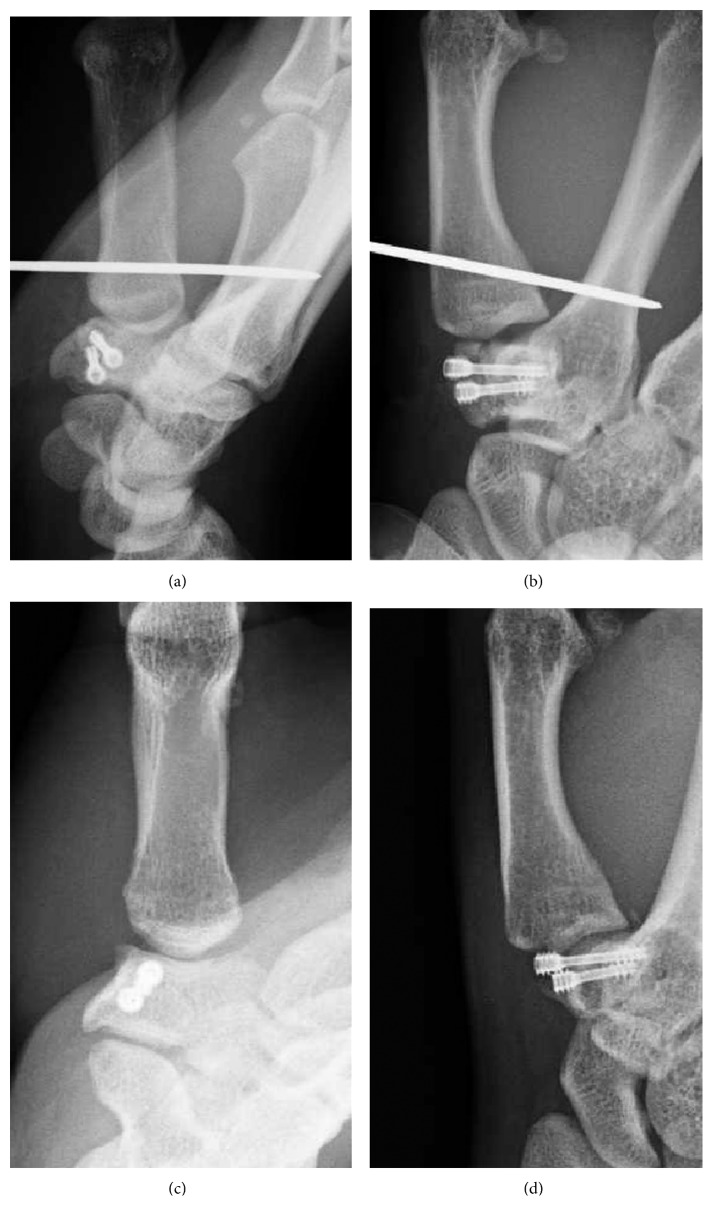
(a, b) Radiographs taken directly after surgery. Good reduction and fixation were obtained using 2 double-thread headless screws. (c, d) Radiographs taken at final follow-up, a year after surgery. A congruent CMC joint and trapezium fracture union is shown. (a, c: anteroposterior view; b, d: lateral view.)
